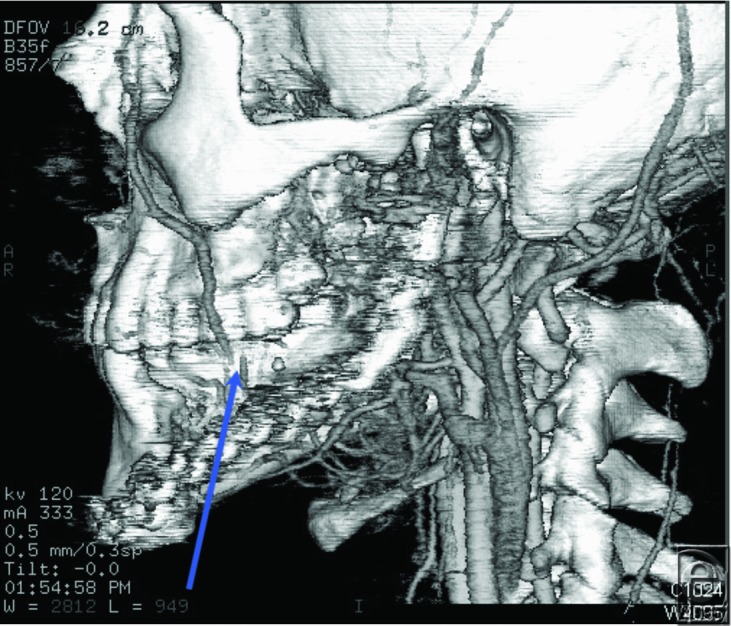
CTA of recipient site for head and neck reconstruction. CTA of head and neck vessels in a patient with a fractured mandibular reconstruction plate. There is total occlusion of the facial artery and significant soft tissue damage near the external carotid artery.
